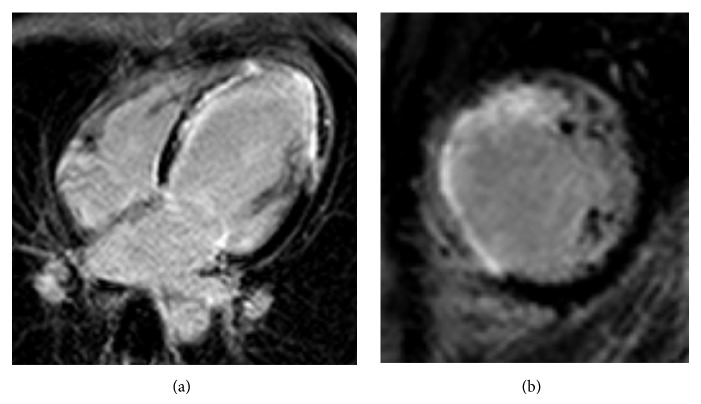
Cardiac MRI. (a) Four-chamber view, obtained 15 minutes after intravenous gadolinium injection. The anterolateral segment shows delayed enhancement in less than 50% of the myocardial thickness. (b) Short-axis view, obtained 15 minutes after intravenous gadolinium injection, showing transmural delayed enhancement of the midcavitary septal segment.
